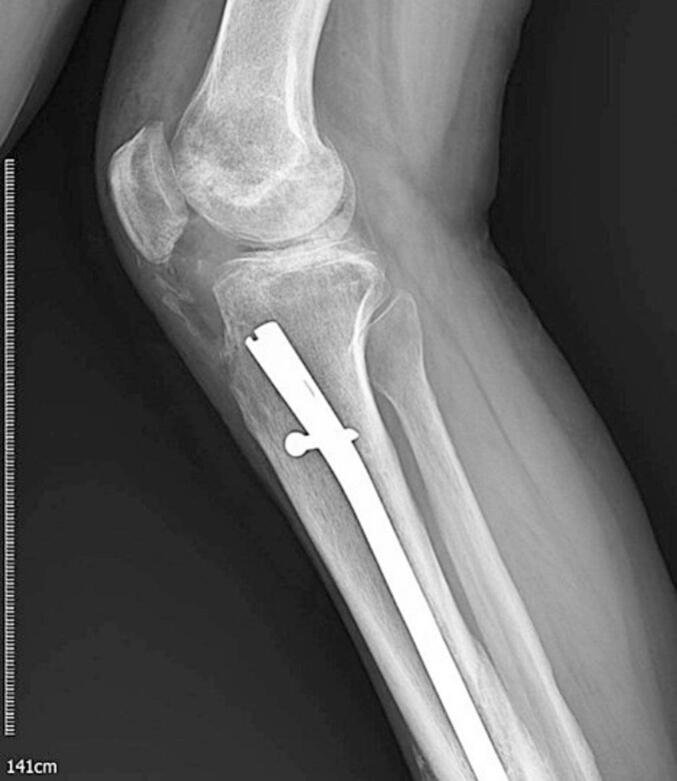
Early postoperative lateral view radiography demonstrates complete HO excision.
